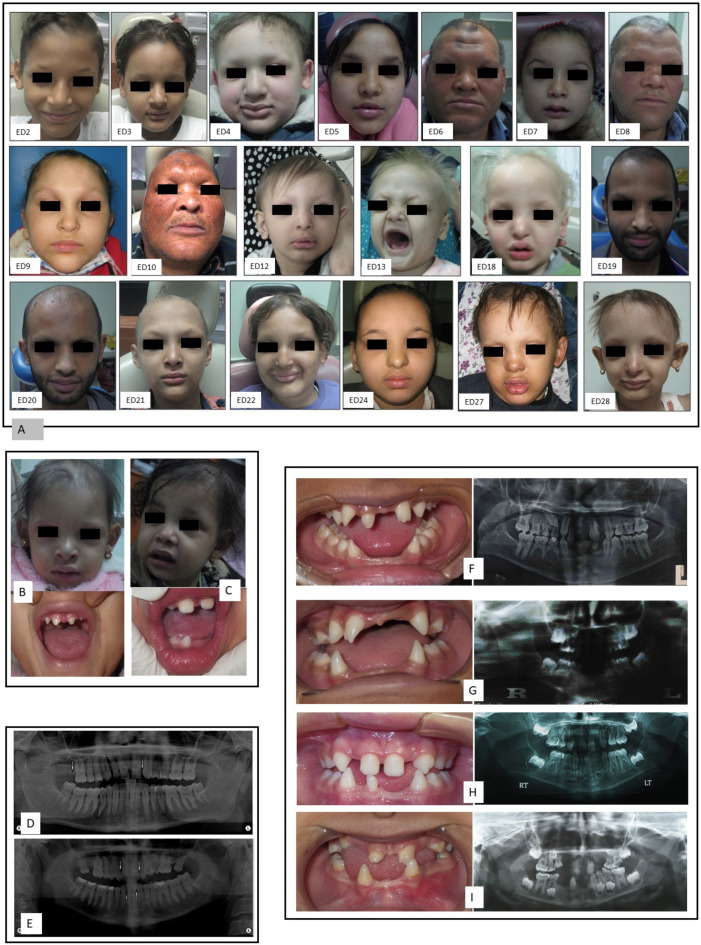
Clinical features and panoramic radiographs of ED patients. (A) Facial photographs of ED patients showing the typical facial features of ED, including sparse hair, thick prominent lips, and depressed nasal bridge. (B,C) Facial and intraoral photographs of ED14 and ED15, respectively, showing the difference between the severely affected ED14 in contrast to milder phenotype of her sister, ED15 of family 8. (D,E) panoramic radiographs of the father and mother of ED28, respectively, showing hypodontia (white arrows indicate sites of missing teeth), which is the only ectodermal feature in these carrier parents, in contrast to the severely affected proband, who showed hypohidrosis, sparse hair without any erupted teeth at the age of 3 years. (F–I) Intraoral photographs and panoramic radiographs of patients ED2, ED3, ED7, and ED24, respectively, showing oligodontia and peg shaped teeth.
